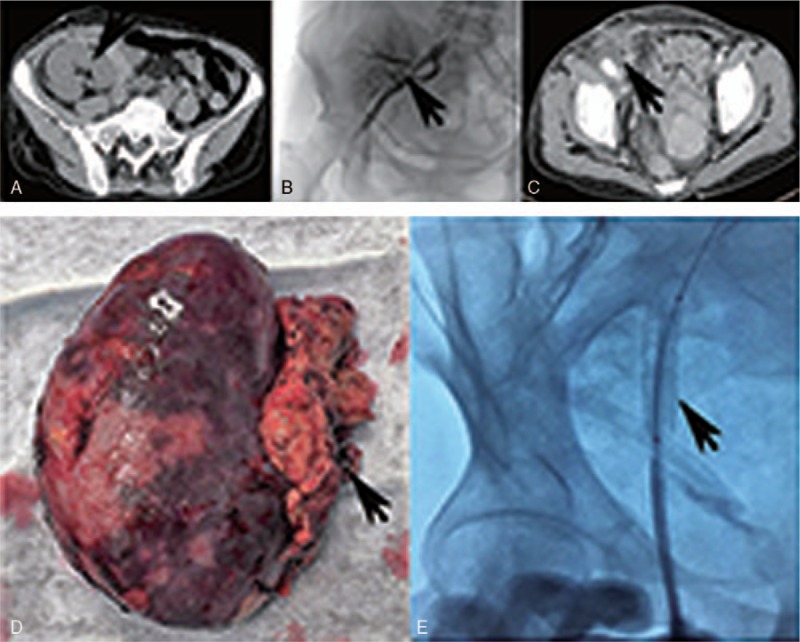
Two renal recipients (recipient 2, 3). (A) CT scan showed massive fluid collection in the perigraft area 14 days after renal transplantation in recipient 2. (B) Iliac artery angiopathy found the bleeding of renal artery anastomosis 14 days after renal transplantation in recipient 2. (C) Enhanced CT scan revealed the right iliac abscess 75 days after resection of renal allograft in recipient 2. (D) Resection renal allograft with corroded artery end 13 days after renal transplantation in recipient 3. (E) Endovascular covered stent placed in the left external iliac artery 13 days after transplantation in recipient 3. CT = computed tomography.
